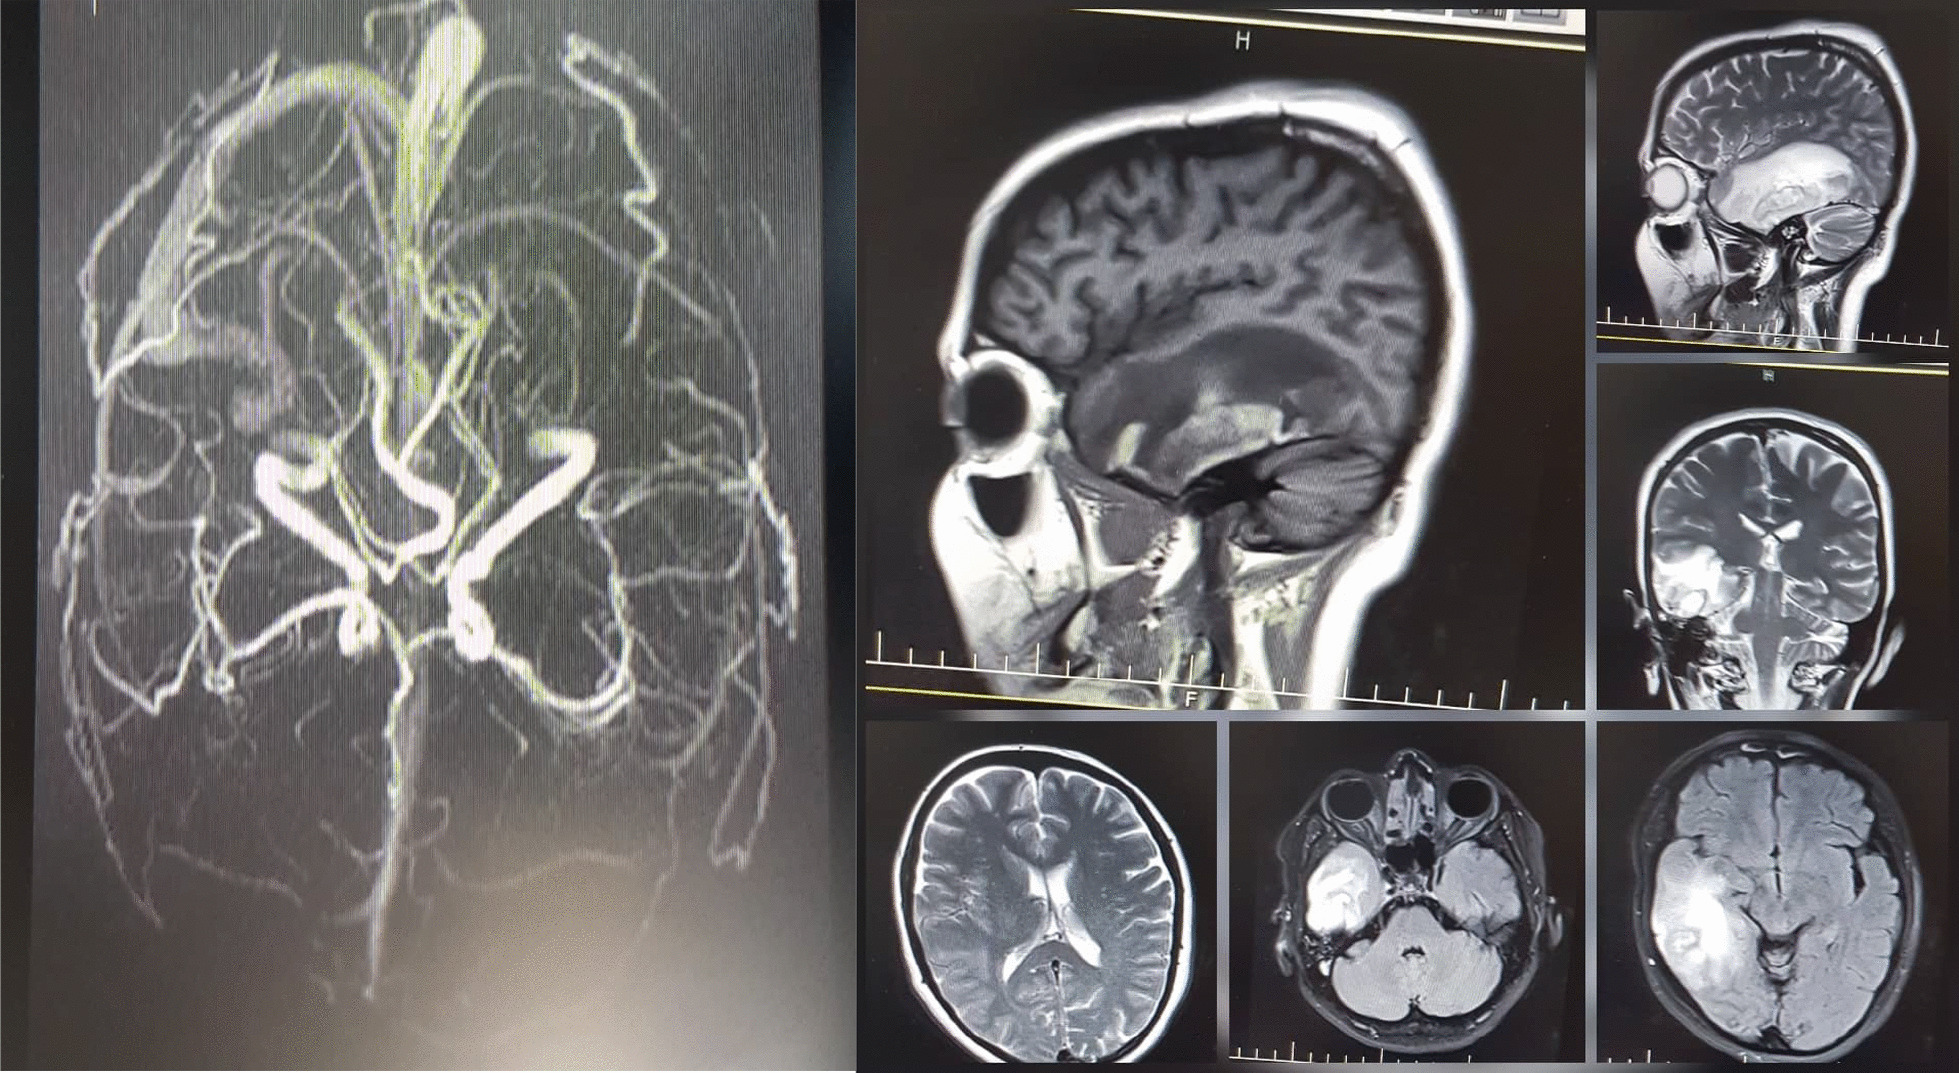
Patient’s brain MRI–MRV. The brain MRI (right) shows a large parenchymal hematoma in the right temporal lobe with peripheral white matter edema with 2 mm of midline shift. The MRV (left) shows engorgement of the right transverse and sigmoid dural sinuses on T2-weighted images and diffuse filling defects compatible with cerebral vein thrombosis
